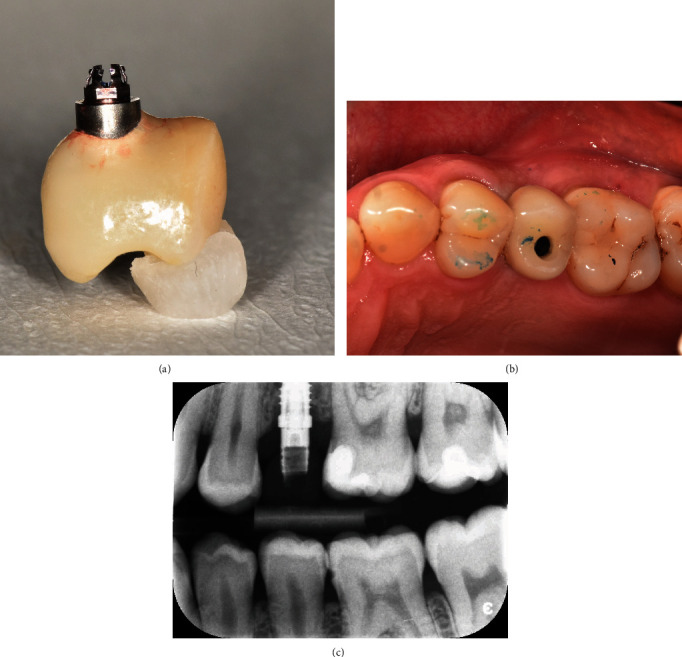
(a) Fabrication of the screw-retained provisional restoration. (b) Occlusal adjustment and installation of the provisional restoration in in situ. (c) Radiograph showed complete seating of the temporary abutment.
